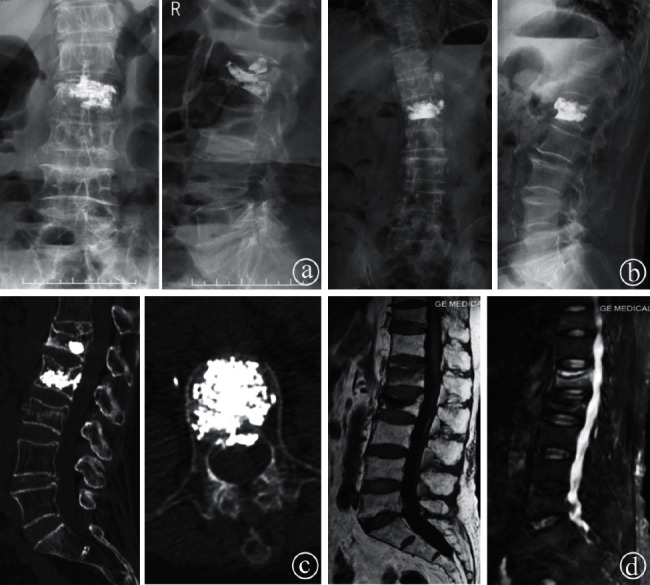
Images of bone cement leakage and adjacent level fracture in PVP cases. X-rays showing that the bone cement had leaked into the intervertebral disk (a). The anterior edge of the vertebral body (b). CT showing that the bone cement had leaked into the vertebral canal (c). MRI scan with compression fracture of the L1 vertebra after PVP of the L2 vertebra (d).
